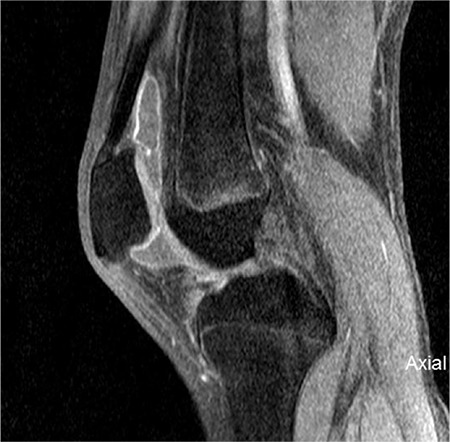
Sagittal T1 fat-saturated weighted magnetic resonance imaging images post contrast injection shows synovial hypertrophy and mild synovial enhancement. Note the amount of synovial fluid
